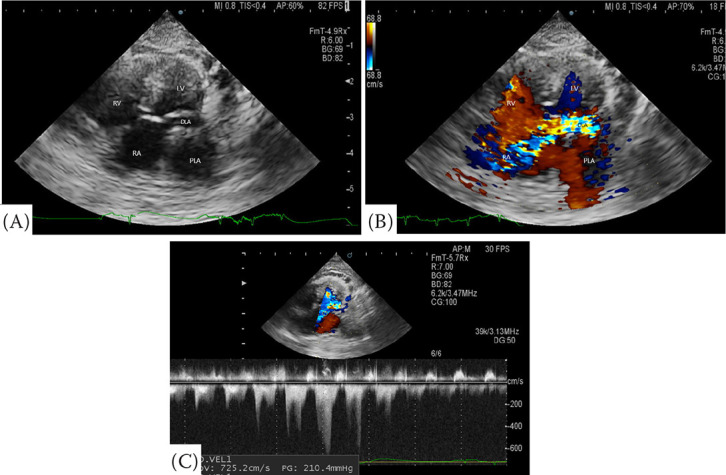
Two-dimensional (A), colour Doppler (B), and continuous wave Doppler (C) echocardiography images in a left-apical view During systole, the flow velocity from the left atrium to the right atrium was measured at 7.2 m/s in a left apical view
